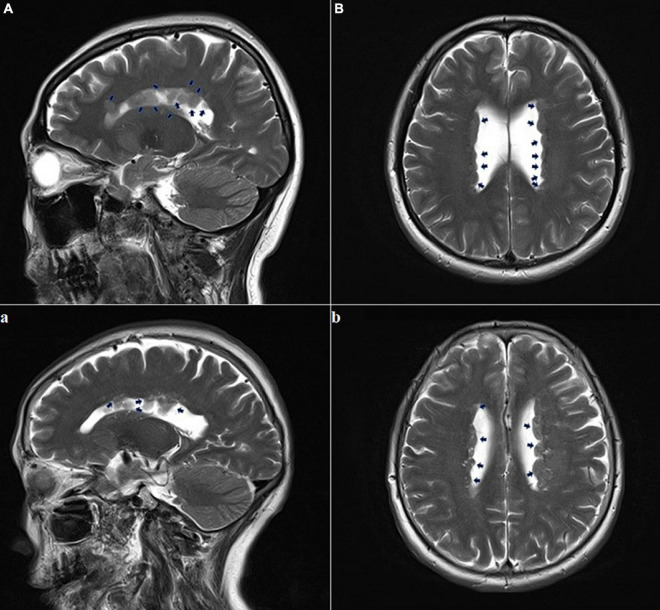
Adult brain MRI images of the pregnant woman and her mother. The pregnant woman (A,B): T2-weighted sequence performed at the age of 35 years showed multiple foci of heterotopic gray matter outlining the walls of both lateral ventricles; this is consistent with the diagnosis of subependymal gray matter heterotopia (black arrows); The pregnant woman’s mother (a,b): T2-weighted sequence performed at 67 years of age showing multiple foci of heterotopic gray matter outlining both lateral ventricles walls (black arrows).
